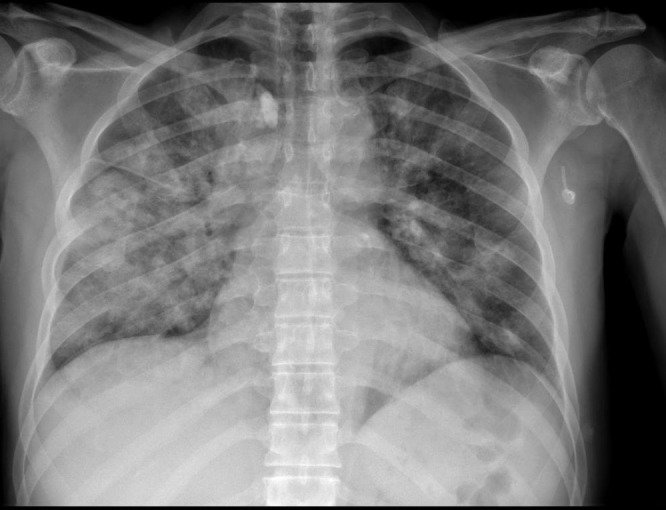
Radiograph the radiologist stated showed positive findings for COVID-19
